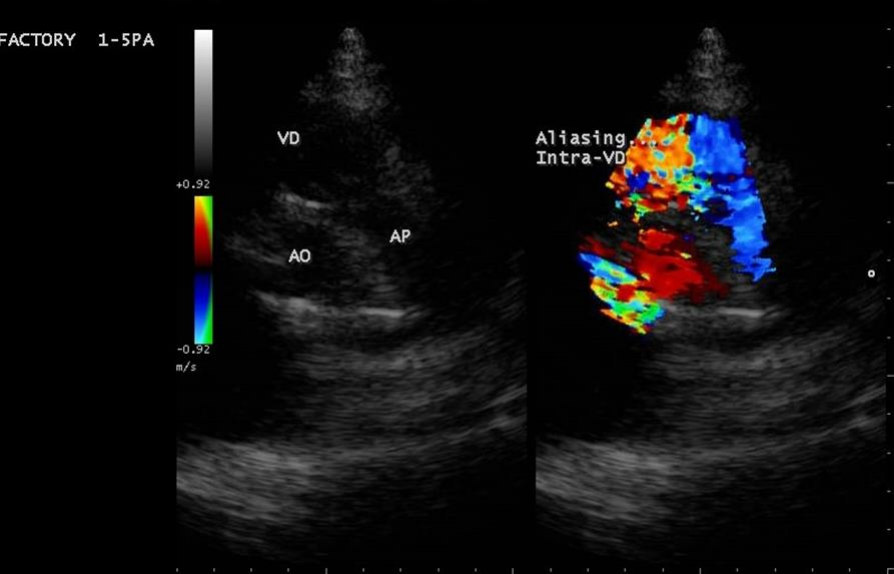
Pré-opératoire: incidence parasternale petit axe, 2D (gauche) et Döppler couleur (droite) avec aliasing témoignant d'une obstruction intra-VD; Ao: aorte; AP: artère pulmonaire
